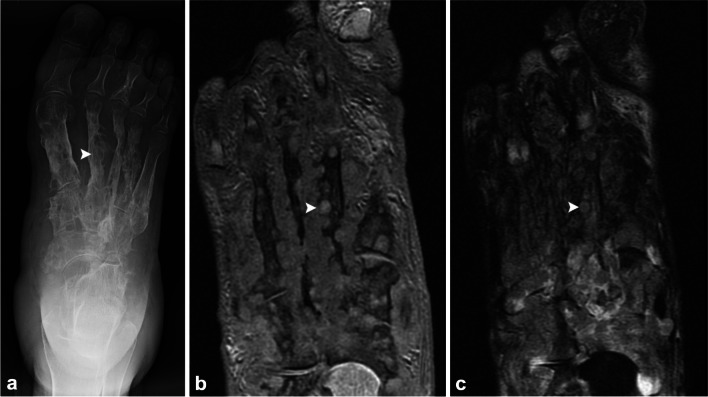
Sixty-year-old woman with chronic right foot pain and infection. AP radiograph (a) and long axis T1 (b) and STIR (c) MRI images of the right foot demonstrate extensive rounded cystic lucencies corresponding to T1 and T2 hyperintense mycetomas with a central T2 hypointense focus (*arrowheads* highlight representative lesion of the second metatarsal), compatible with the “dot-in-circle” sign in this patient with fungal osteomyelitis (Madura foot)
